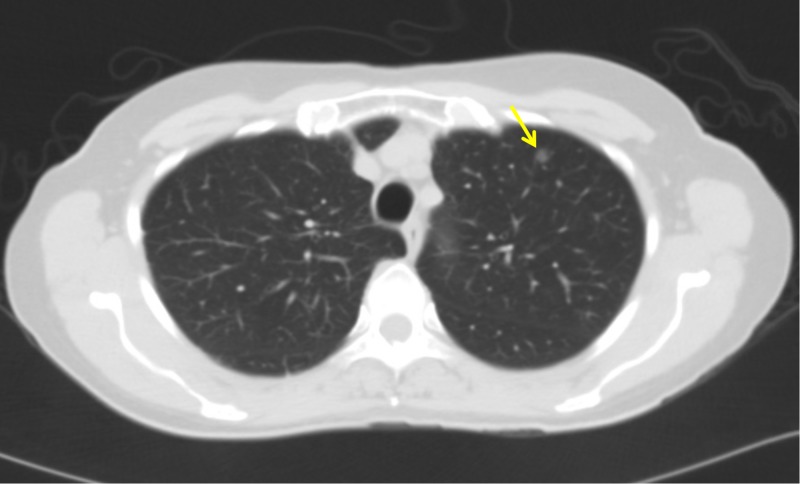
CT scan of the chest demonstrating a 6 mm left upper lobe pulmonary nodule (arrow)
